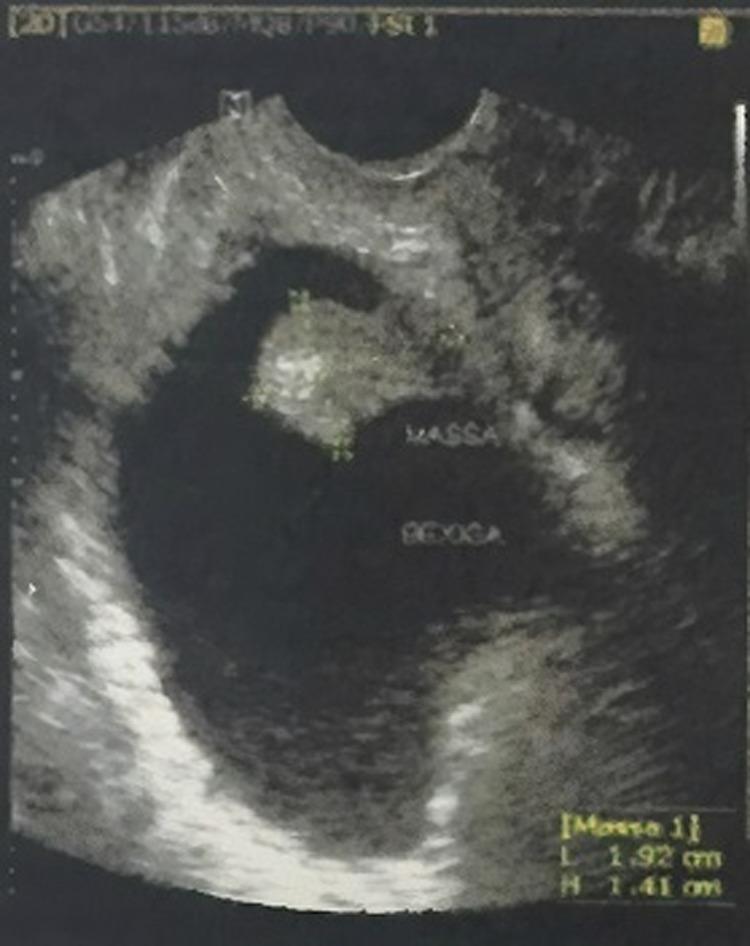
Transvaginal pelvic ultrasonography revealing hyperechogenic nodule.
